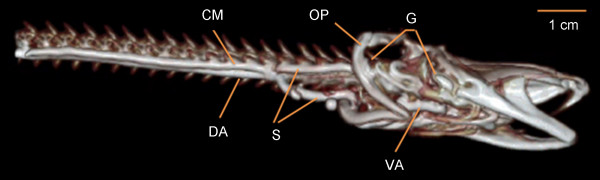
CTA of *Monopterus albus *using Microfil contrast medium showing a lateral view of the head region; anterior to the right. Image created by 3D rendering software following CT. Key: VA, ventral aorta; DA, dorsal aorta; S, shunt vessels joining ventral and dorsal aorta; CM, celiacomesenteric artery; G, right gill region; OP, right operculum.
